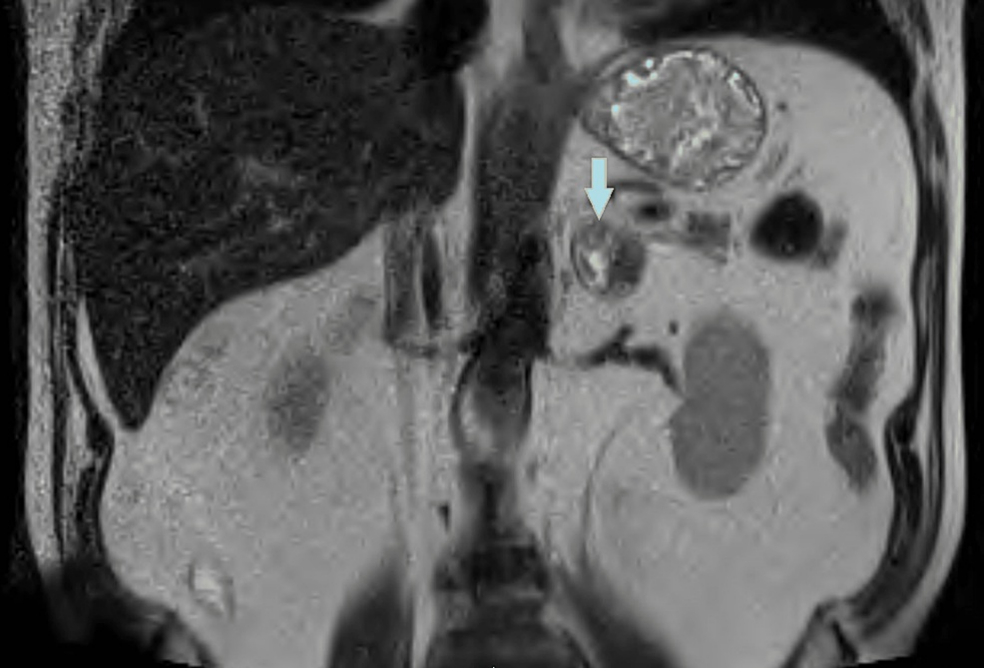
MRI of the abdomen (coronal section) showing left adrenal gland hemorrhage (Blue arrow with yellow margin).
